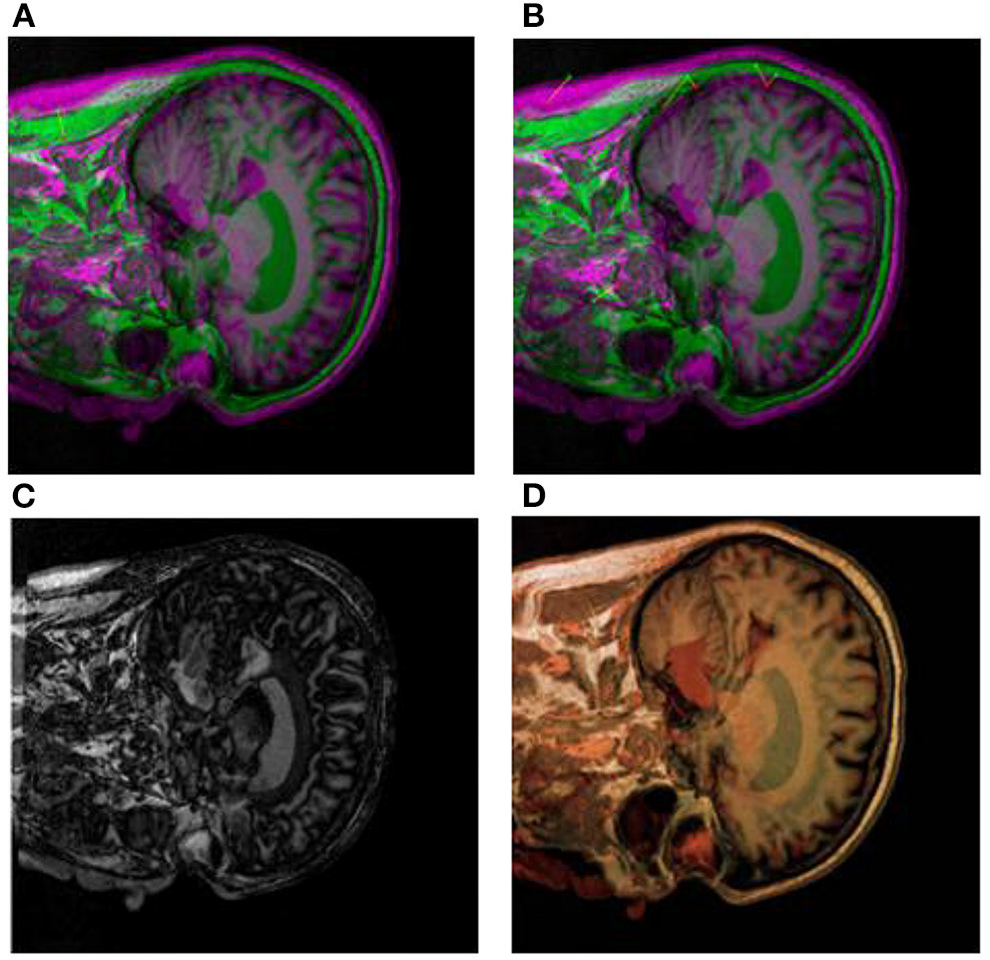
Segmental registration images of brain CT-MRI and PET images. (A) shows the pre-processed initial registration image. (B) medical images after multi-modal registration. (C) is affine transformation global rough registration of medical images. (D) represents the medical image after non-rigid transformation and high-precision registration, and the addition of false color is convenient for physicians to identify.
